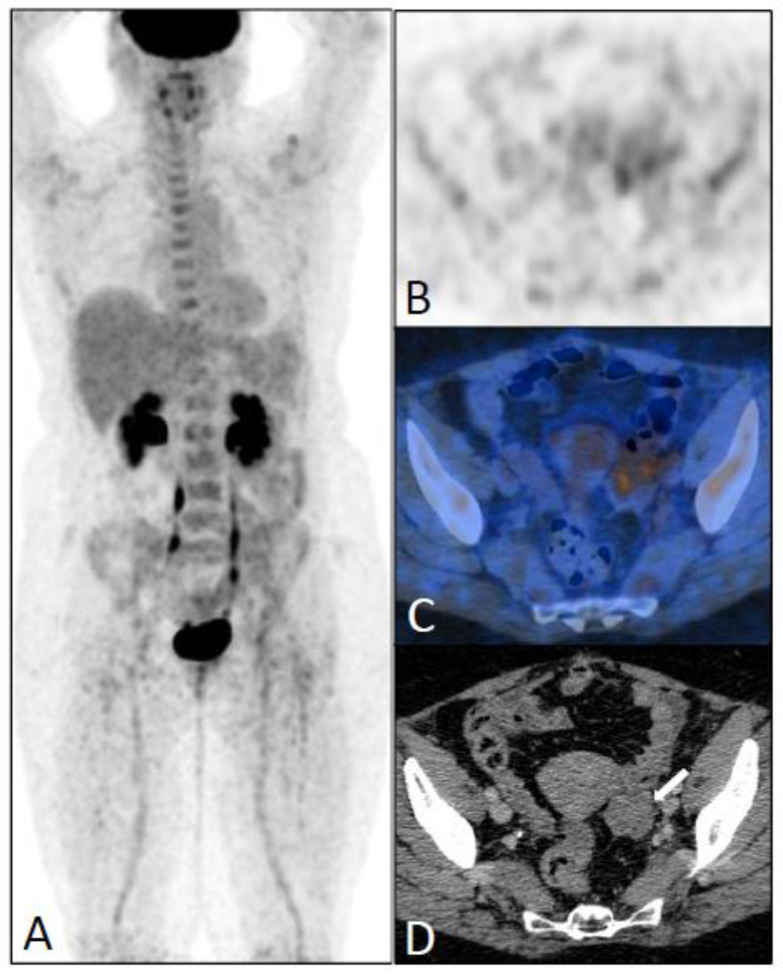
An FDG-PET/CT scan performed eight years after the initial diagnosis of ILC (pT3N1, SBR II) in a 55-year-old patient, due to elevated tumor marker (CA 15.3 = 249). Maximum intensity projection (A), Axial PET (B), and PET/CT fusion (C) images showing a faintly increased FDG uptake not described in the report. A left uterine mass (white arrow) associated with a peritoneal effusion was found on a CT scan (D) performed one week after the PET/CT scan. Peritoneal and ovarian metastases were confirmed by laparoscopy and chemotherapy was introduced. This patient died from disease progression in the peritoneum nine years after the diagnosis of recurrence.
